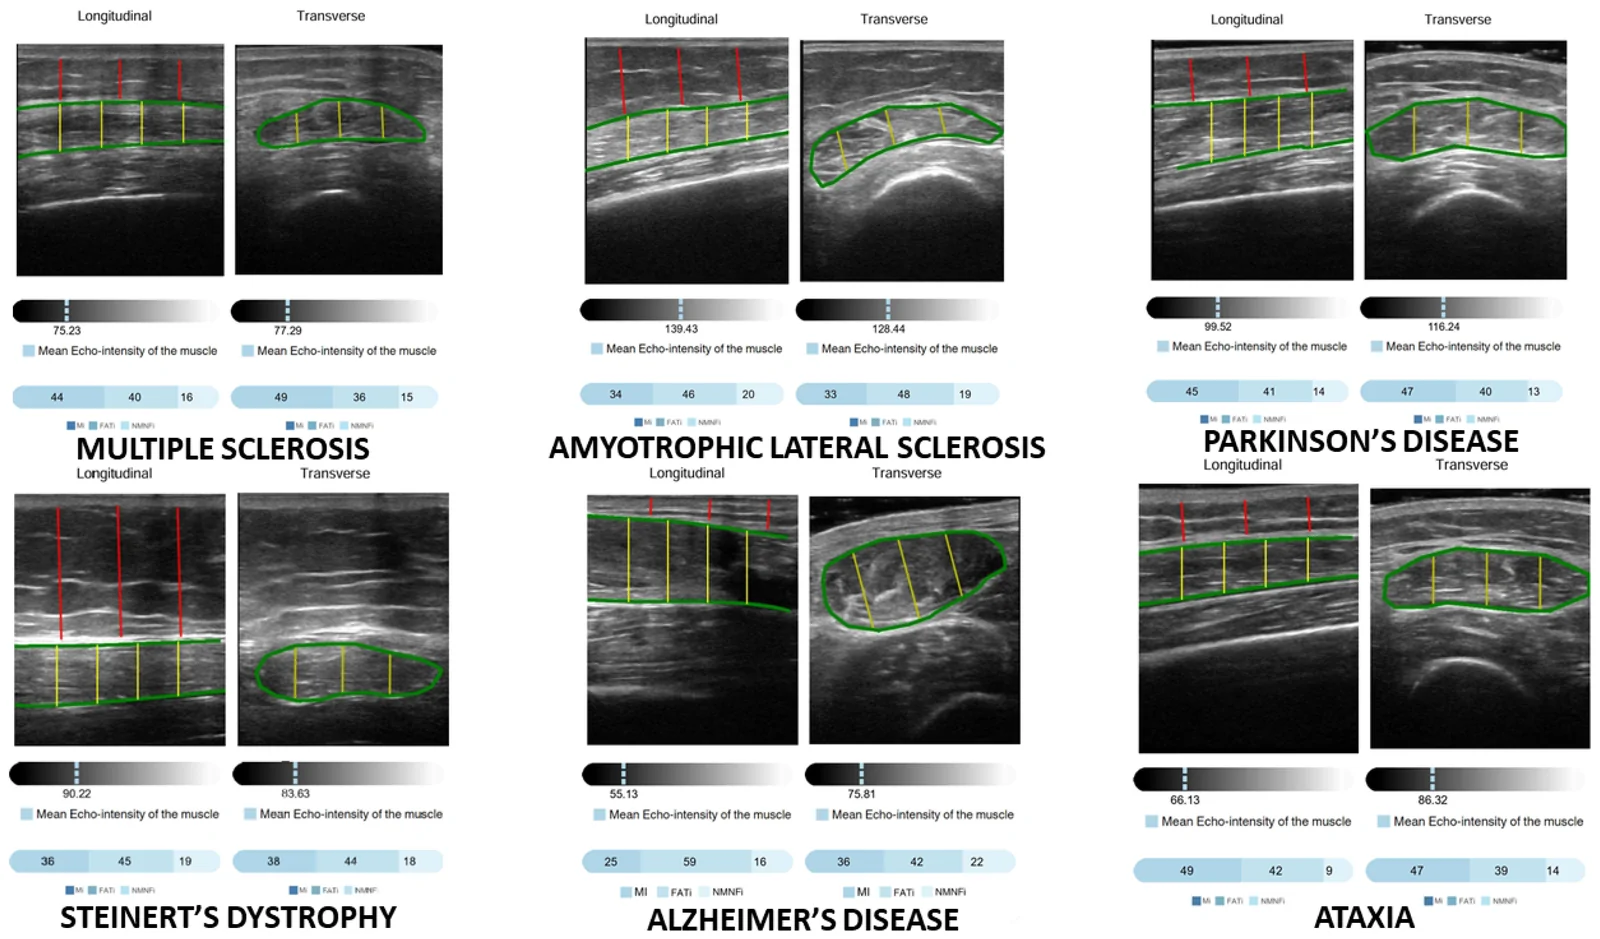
Different rectus femoris ultrasound images analyzed by Artificial Intelligence tool (PIIXMEDTM). Mi: Low Echogenicity Index (Muscle Index); FATi: Medium Echogenicity Index (Fat Index); NMNFi: High Echogenicity Index (No Muscle No Fat index). Red line: adipose tissue, green line: muscle fascia; yellow line: muscle thickness.
